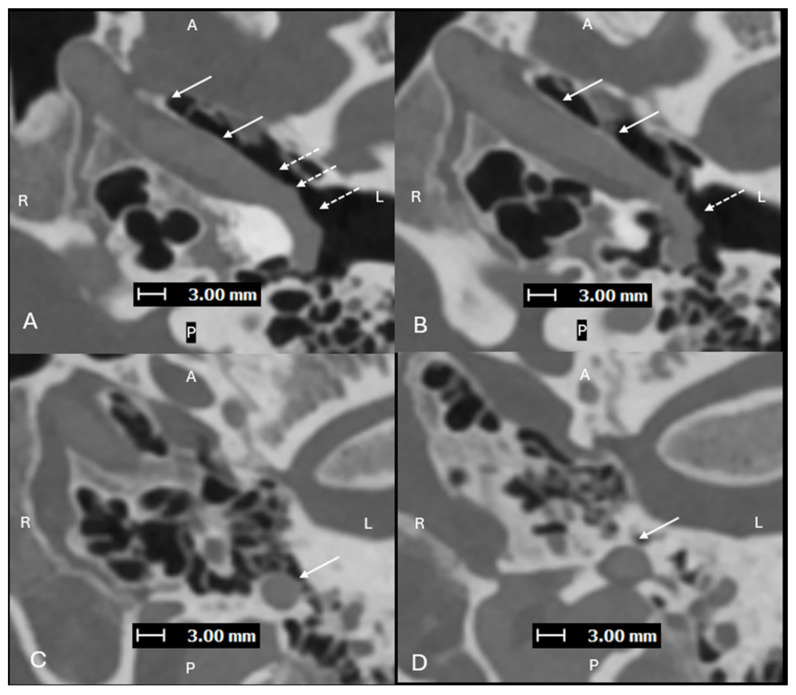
Axial images (superior to inferior (A–D), with A, P, L, R for orientation donating anterior, posterior, left, and right respectively) from a PCD-CTA study—with UHR mode (Collimation 120 × 0.2 mm), Tube voltage (120 kVp), slice thickness/increment (0.2/0.2 mm), and sharp Kernel (Hv56) with Quantum Iterative Reconstruction (3)—demonstrating an aberrant L ICA (solid arrows) within an enlarged ITC (dashed arrows). Aberrant ICA are congenital vascular anomalies that occur from the involution of the cervical portion of the ICA, which leads to enlargement of the normally small collateral inferior tympanic and caroticotympanic (hyoid) arteries [1]. The segment most noted as the aberrant ICA is the inferior tympanic artery [2]. The inferior tympanic artery is a branch of the external carotid artery, usually the ascending pharyngeal artery, which extends through the inferior tympanic canaliculus (ITC), and is enlarged in the setting of an aberrant ICA [3]. As this artery courses along the cochlear promontory (via the ITC), no carotid plate separates the vessel from the middle ear cavity, and the vessel appears as a vascular retrotympanic mass on exam. It is very important to recognize an aberrant ICA on imaging. Accurately describing the presence of this aberrant vessel is important to help prevent iatrogenic injury during intervention of the middle ear. While typically asymptomatic in some cases, it can present with hearing loss and sometimes pain [4,5]. An aberrant ICA is commonly included in the differential diagnosis for vascular middle ear masses with other entities such as lateralized ICA, dehiscent jugular bulb, aneurysm of the petrous ICA, glomus tympanicum tumors, and glomus jugulare tumors [6,7].
